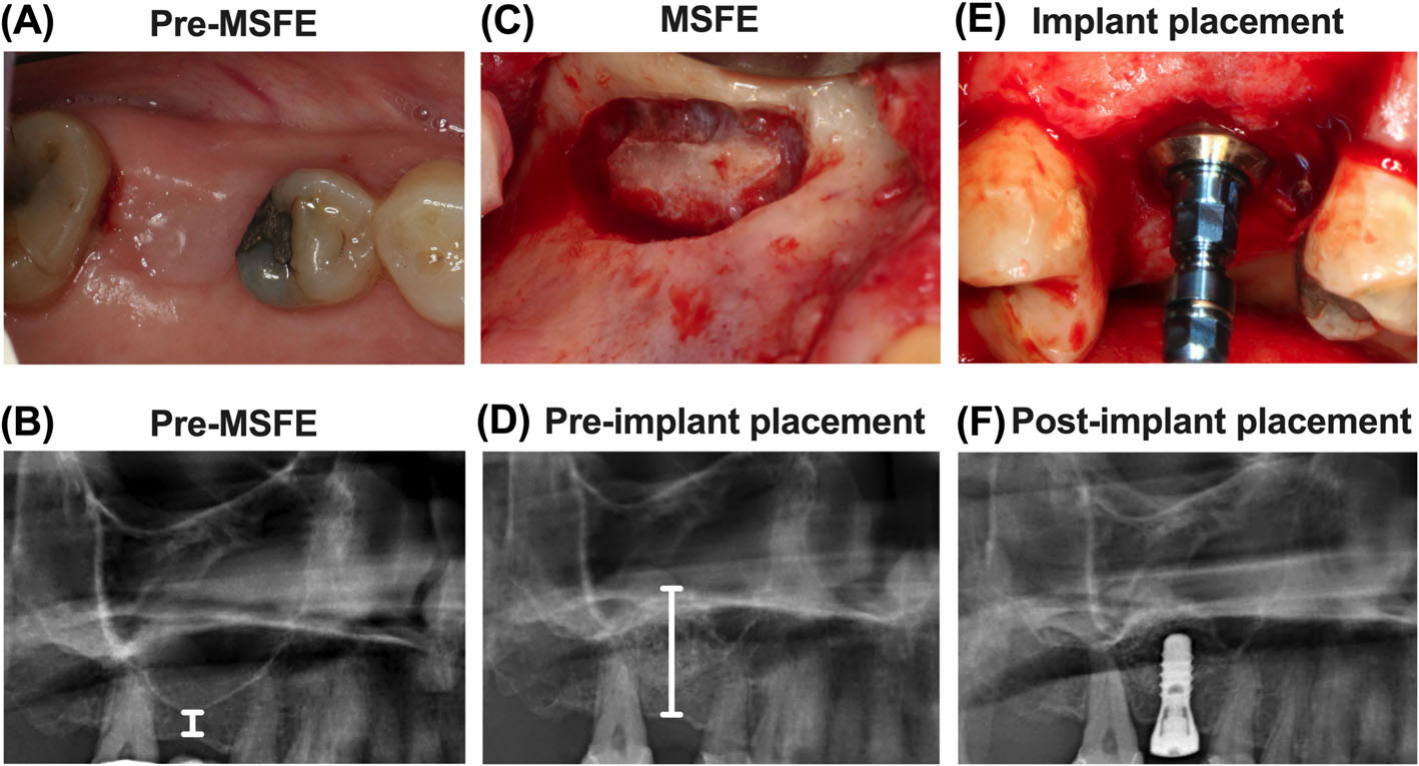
Clinical photographs of maxillary sinus floor elevation (MSFE) via a lateral approach allowing dental implant placement, and their corresponding radiographs to evaluate maxillary sinus and alveolar bone height. (A) Preoperative photograph of the clinical situation. (B) Preoperative radiograph of the maxillary sinus. White line: residual native bone height. (C) Photograph of the lateral window during MSFE. (D) Radiograph of the maxillary sinus 4 months post‐MSFE prior to implant placement. White line: total bone height. (E) Photograph of implant placement at 4 months post‐MSFE. (F) Radiograph of the maxillary sinus directly after implant placement.
